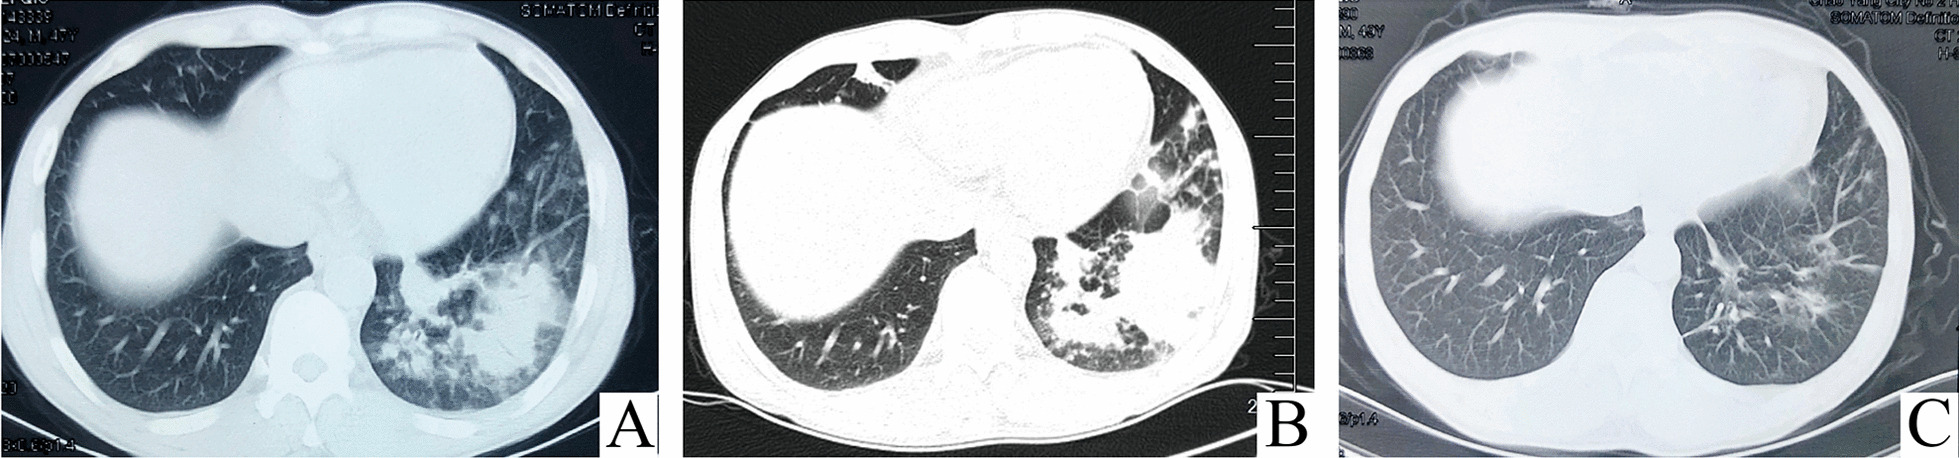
Computed tomography (CT) of the patient’s chest showing progression of the pulmonary lesions. a Chest CT performed at a local hospital prior to admission to our hospital showing irregular patchy opacities in the left lower lobe. b Chest CT performed on admission to our hospital showing extension of the patchy opacities in the left lower lobe since the previous scan, and new small patchy opacities in the right middle lobe. c Follow-up chest CT performed after 1 month of corticosteroid treatment showing almost complete resolution of the pulmonary lesions
